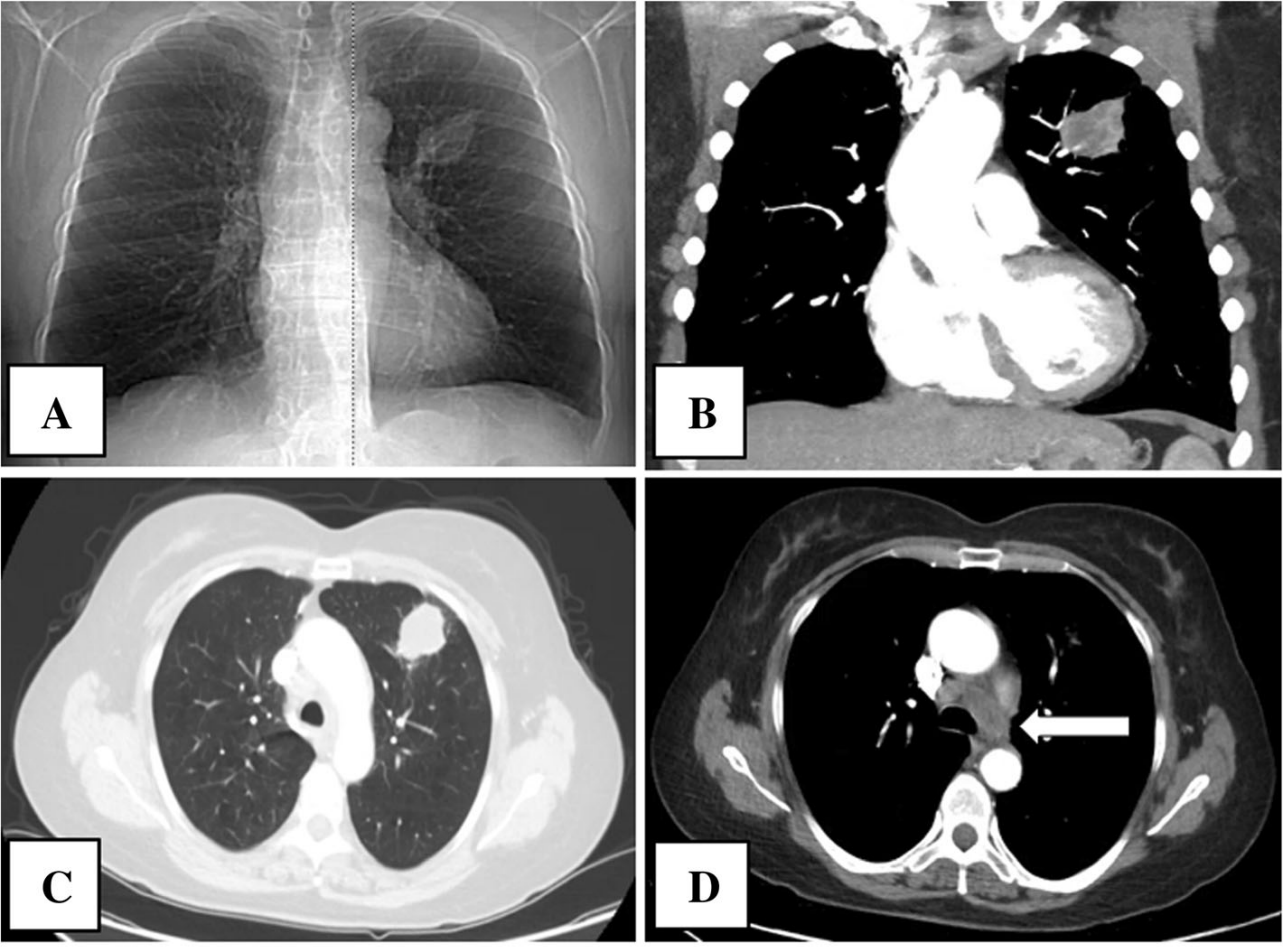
Chest X-ray and computed tomography showed a tumor in the left lung field. a CXR showed a round mass in the left upper lung field. b CT coronal image demonstrated the mass anteriorly within the left upper lobe. c CT scan revealed a 3.4 × 3.1 cm left upper lobe pulmonary mass lesion most compatible with primary lung cancer. d CT scan showed abnormal left hilar and mediastinal adenopathy (*arrow*) suggestive of metastatic nodal involvement
